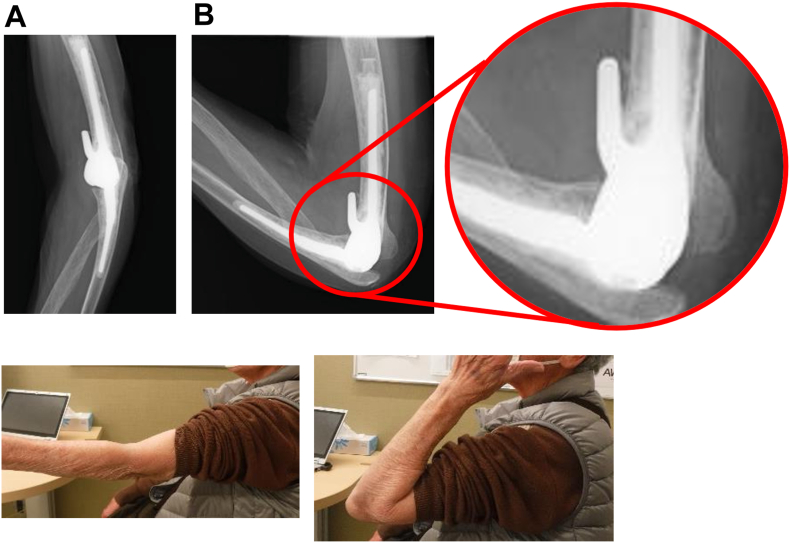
(A) Plain radiograph of the elbow in maximum extension. (B) Plain radiograph of the elbow in maximum flexion showing no impingement between the anterior flange and the coronoid process.
